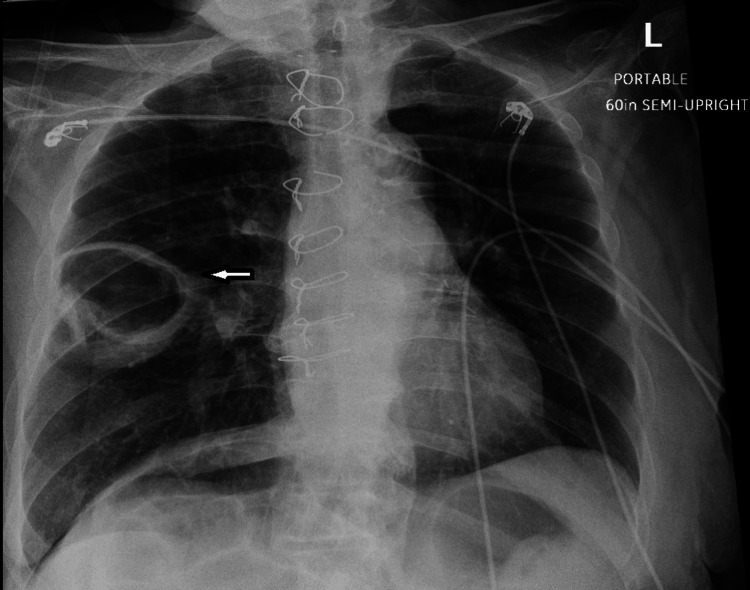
Chest radiograph obtained at subsequent admission demonstrating a new smooth-walled cavitary lesion in the right middle lobe, in the prior area of consolidation.
